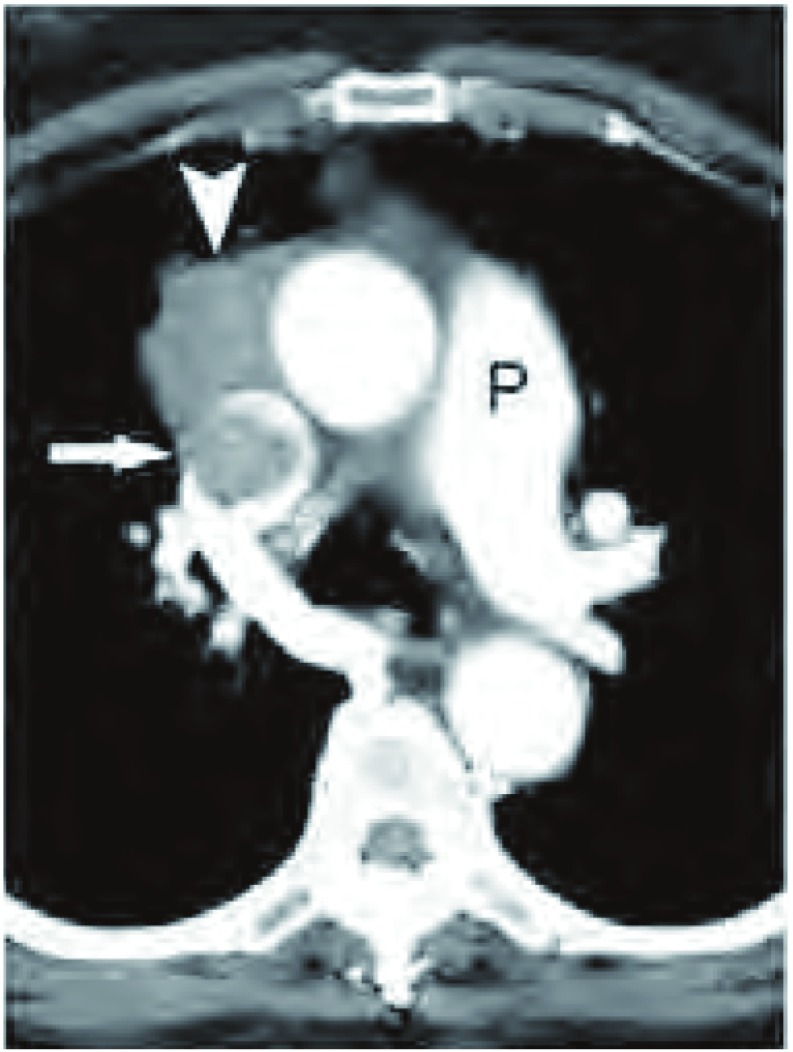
紧邻血管。胸部增强CT（软组织窗）所示，胸腺瘤（T）几乎完全包绕头臂静脉（长箭头所示），而与升主动脉（A）的接触面为30%。短箭头所示为增强的并行静脉。 Abutment of vessels. Contrast-enhanced chest. (CT) (soft tissue window) demonstrates the thymoma (T) nearly completely encasing the right brachiocephalic vein (white arrow), whereas only abutting about 30% of the aorta (A) circumference. Arrowhead: contrast filled collateral vein.
